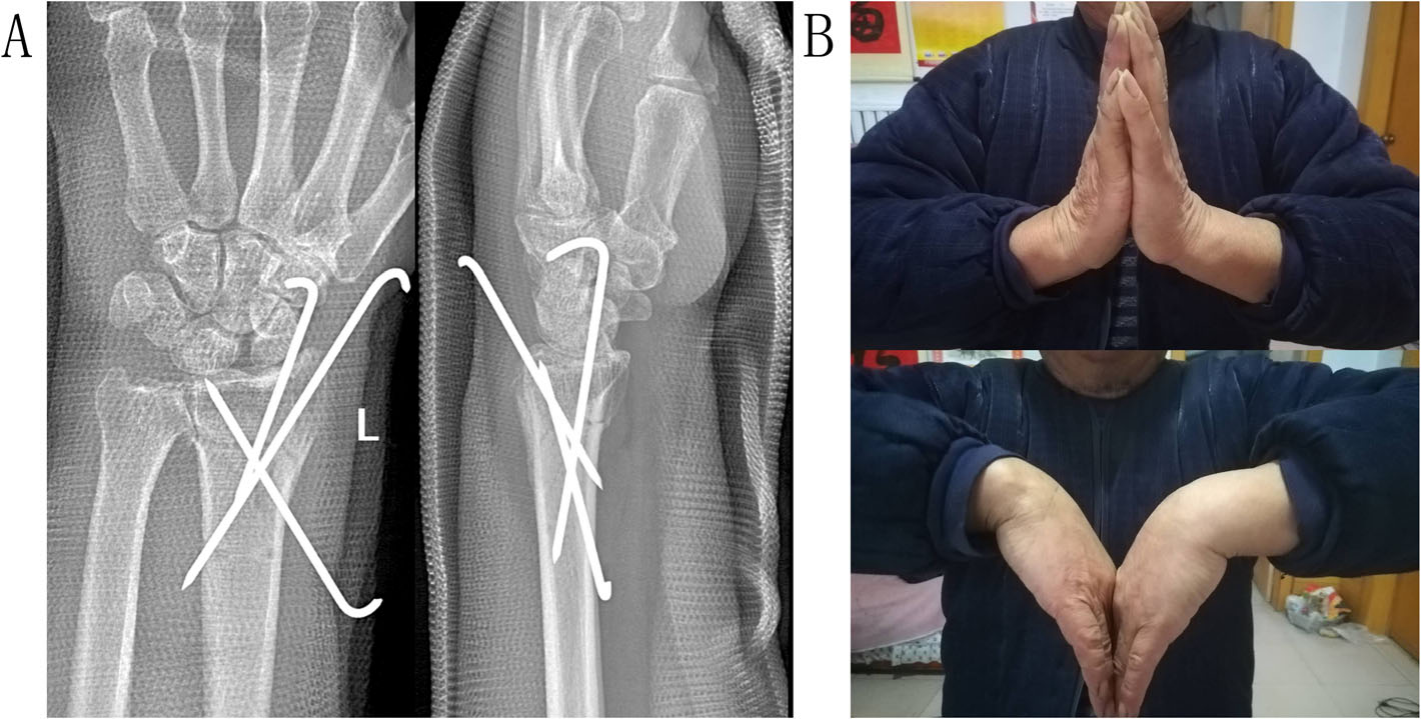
The frontal and lateral X-ray films (A) showed evidence of the excellent condition of bridging in the fracture end 6 weeks postoperative. Six months postoperative, the affected wrist achieved a good function (B). The VAS score was 0, the modified Mayo wrist score was 80, and the *Quick*DASH score was 15.99
